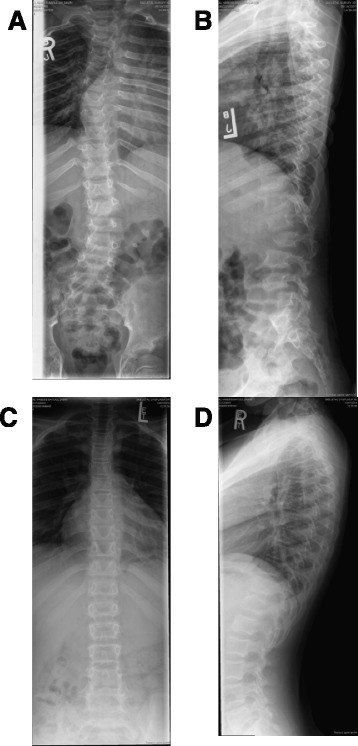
Spinal radiographs from sibship 2 patients. Panel a and b show radiographs of the older sibling at age 8, after 5 years of ERT. Note the ‘S’ shaped scoliosis with right-sided convexity in the thoracic region and left-sided convexity in the lumbar region as well as gibbus deformity at L1 and L4. There is retrolisthesis of L4 with respect to L5 and possible compromise in the spinal canal at L1 and L4. Small anterior beaking is noted in the lower thoracic and lumbar vertebrae. Panel c and d are spinal radiographs of the younger sibling at age 10, after 10 years of ERT. Note the absence of spinal deformity with only minimal anterior beaking of the lower thoracolumbar vertebrae
